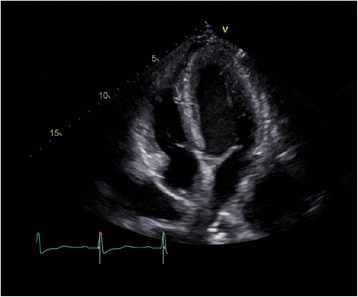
Transthoracic echocardiography, after 6 days of corticosteroid treatment; apical four-chamber view showing marked regression of the myocardial thickening previously seen
